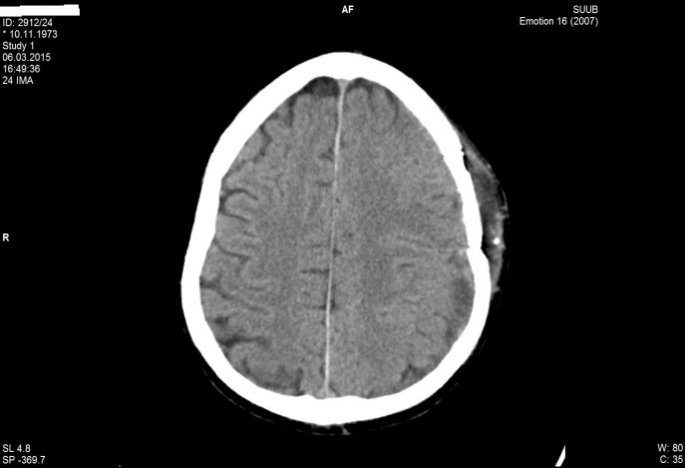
Postoperatory CT aspect in patient M.F. of 41 years old
